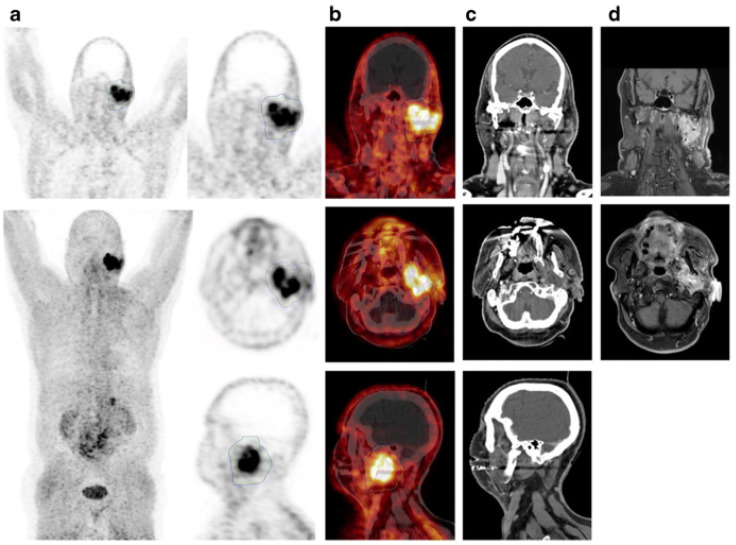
PET imaging with ^68^Ga-FAPI is superior to conventional MRI scan. (A, B) A patient receiving a PET scan with ^68^Ga-FAPI to noninvasively image a mucoepidermoid carcinoma lesion on the left parotid gland. PET images show tracer uptake at the tumor site with low background. (C, D) Conventional MRI imaging shows diffuse tumor infiltration, thereby making delineation of tumor and stromal tissue difficult and subjective. (Adapted for use under Creative Commons license http://creativecommons.org/licenses/by/4.0/ from Syed, M., Flechsig, P., Liermann, J., *et al.* Fibroblast activation protein inhibitor (FAPI) PET for diagnostics and advanced targeted radiotherapy in head and neck cancers. *Eur J Nucl Med Mol Imaging*
